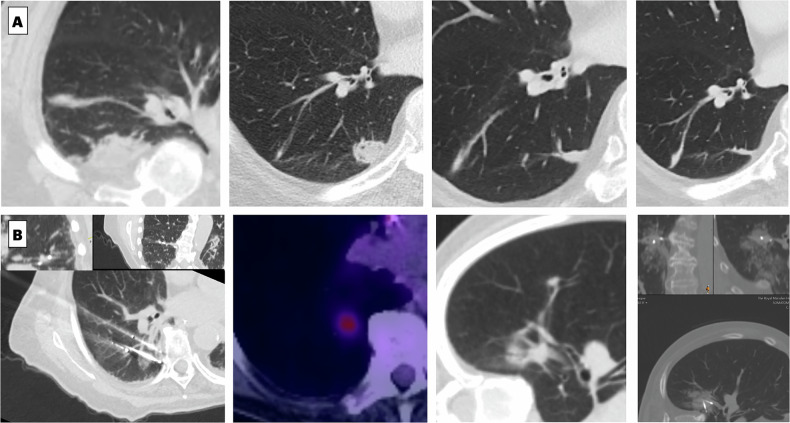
Examples of response to robot-assisted cryoablation. A Durable response to cryoablation. A 76-year-old man underwent ablation of a right lower-lobe rectal cancer metastasis. Serial CT images demonstrate involution of the ablation zone over time: immediately post-ablation, at 6 weeks, 4.5 months, and 11 months, without evidence of recurrence. B Local tumour progression following cryoablation. A 79-year-old woman with colorectal metastases underwent ablation of a right lower-lobe tumour. At the time, needle placement appeared satisfactory; however, in hindsight, the superior-inferior coverage was likely insufficient, which highlights the need to carefully review multiplanar reformats during needle placement. The FDG PET/CT 8.3 months post-ablation showed medial recurrence, subsequently treated by repeat cryoablation. Follow-up is ongoing
